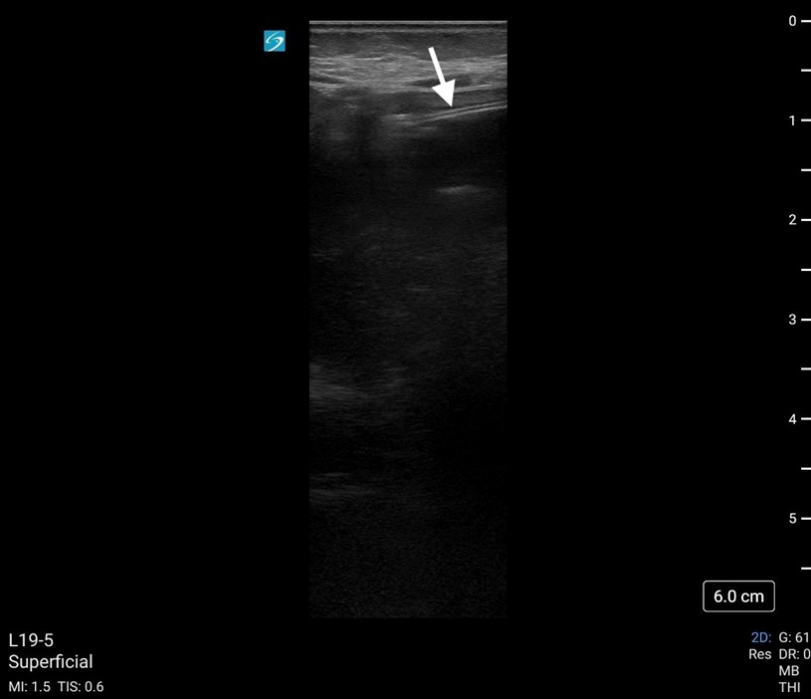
Enteral feeding tube (white arrow) demonstrated in antrum of stomach using point of care ultrasound (POCUS). Feeding tube visualized as 2 parallel hyperechoic lines.
